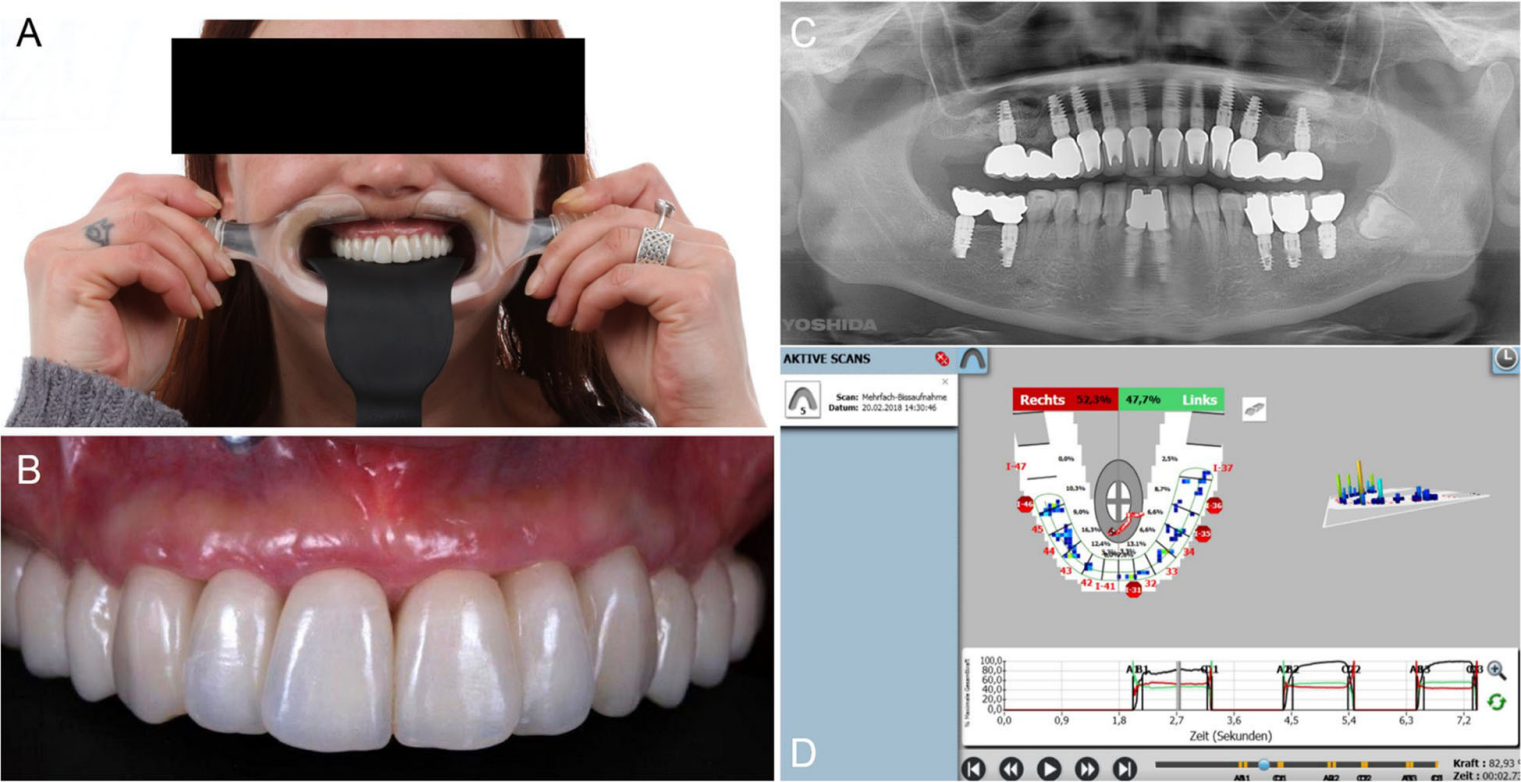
Delivery of the final restorations. a Healthy and aesthetic aspect of the restorations. b Intraoral picture. c Final panoramic radiograph. d Occlusal control with Tekscan®. The stability of the occlusal situation was checked after delivery and in the recall sessions, at least once a year
